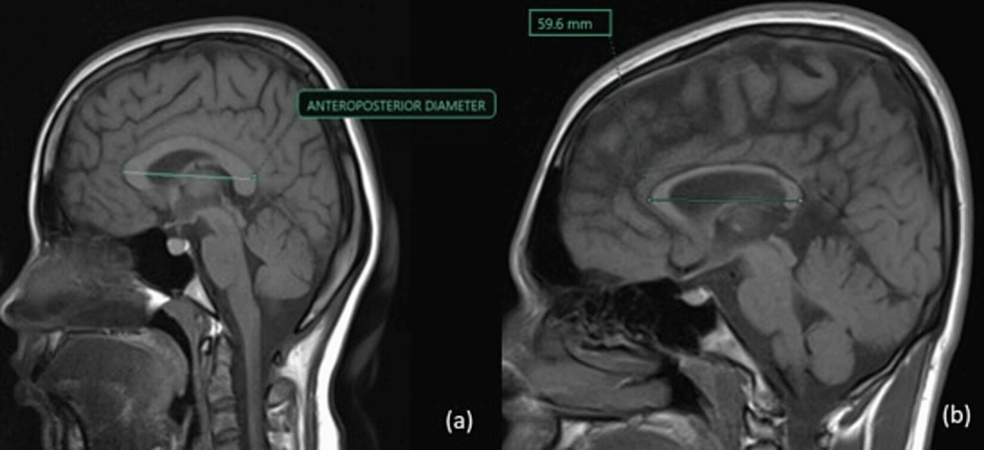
Mid Sagittal T1W magnetic resonance images showing the measurement of the anteroposterior diameter of the corpus callosum Measurement of the anteroposterior diameter of the corpus callosum at its maximum level in control (a) and reduced in case (b).
